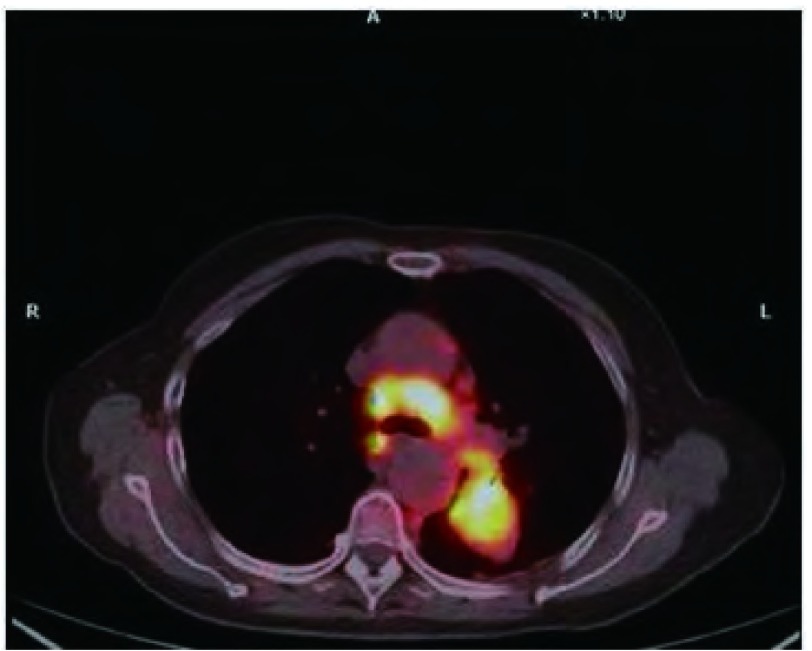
PET-CT示：纵隔内多发大小不等淋巴结，代谢异常增高，左肺上叶软组织肿块影，代谢异常增高 PET-CT scan revealed the enlargement of mediastinal lymph nodes was in multiple sizes, with higher uptake of FDG. The mass on the left upper lobule of the lung also showed higher uptake of FDG
